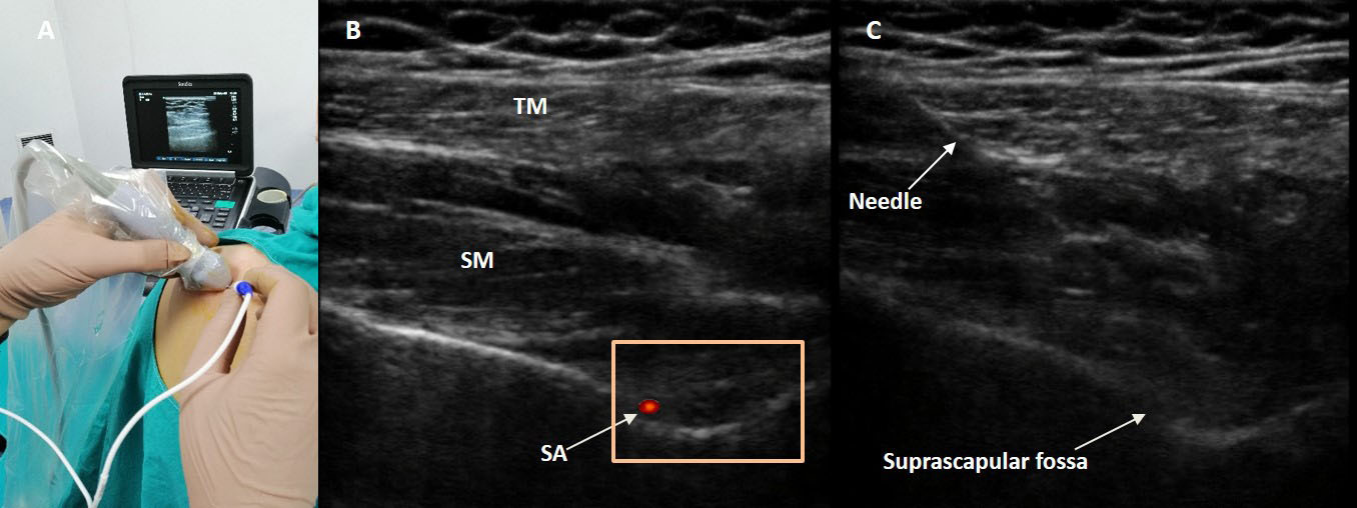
A) The positioning of the linear ultrasound transducer and radiofrequency electrode. B) Scanning of the suprascapular nerve with linear ultrasound probe; trapezius muscle (TM), suprascapular muscle (SM), suprascapular notch, and color Doppler imaging of the suprascapular artery (SA). C) Real-time imaging of the needle insertion under ultrasonographic guidance.
